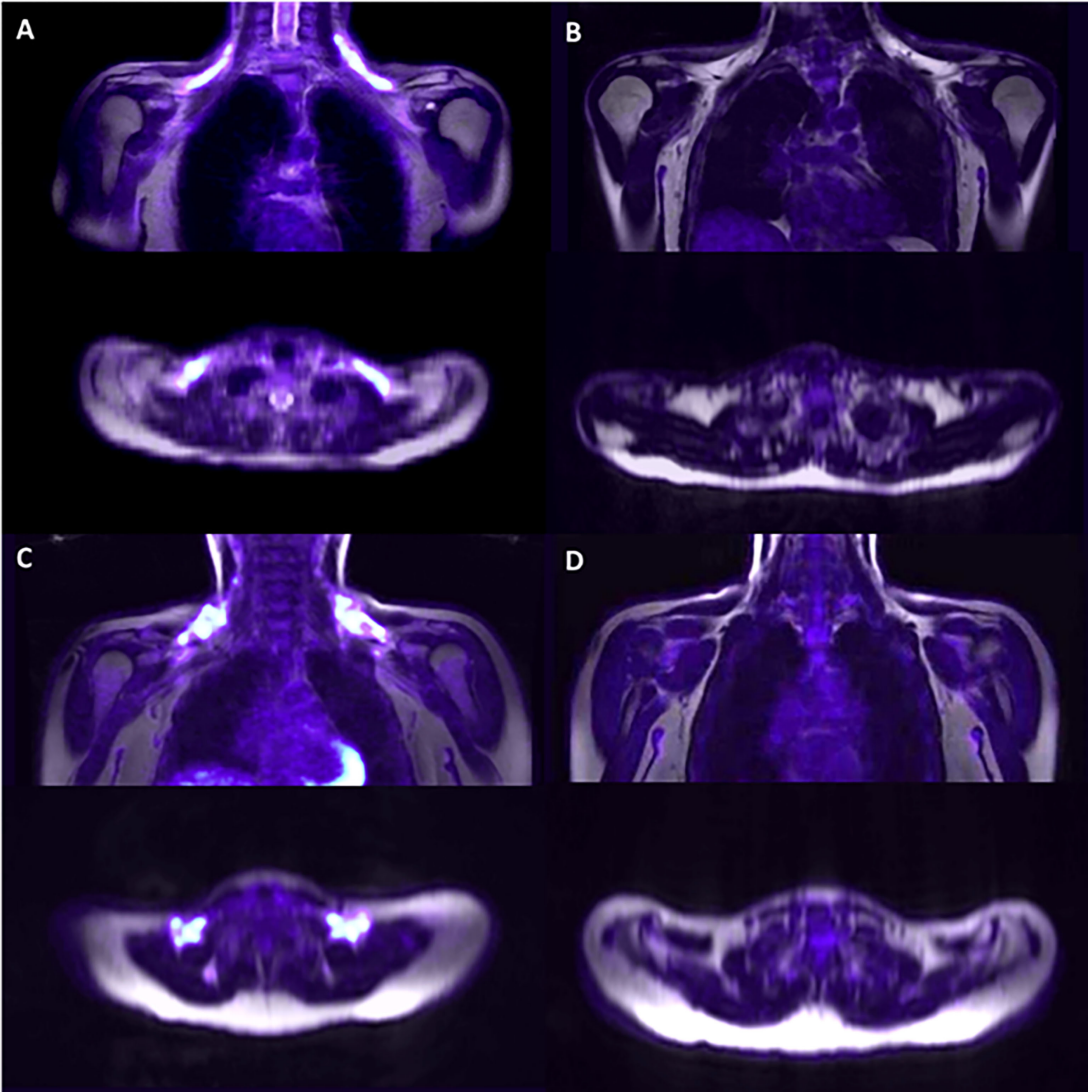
Representative PET/MRI of the supraclavicular area after cold exposure. Supraclavicular area of lean subjects with detectable BAT (A) and non-detectable BAT (B) and subjects with overweight/obesity with detectable BAT (C) and non-detectable BAT (D).
